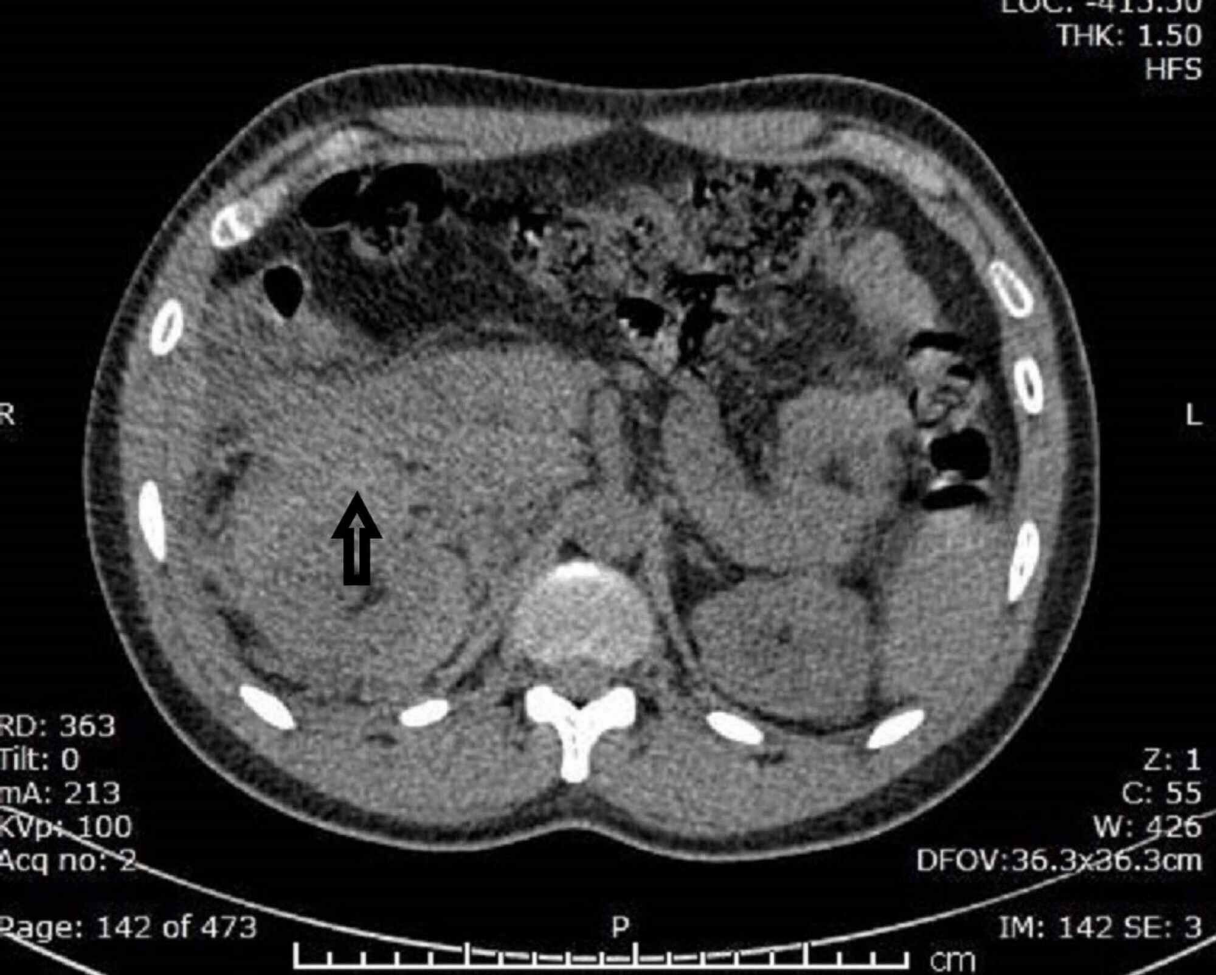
Noncontrast CT-KUB showing enlarged right kidney with perinephric fat stranding and isodense lesion in the perinephric space (arrow), showing a CT density of recent bleed (45-50 HU). CT-KUB, computed tomography-kidney ureter bladder; HU, Hounsfield unit
